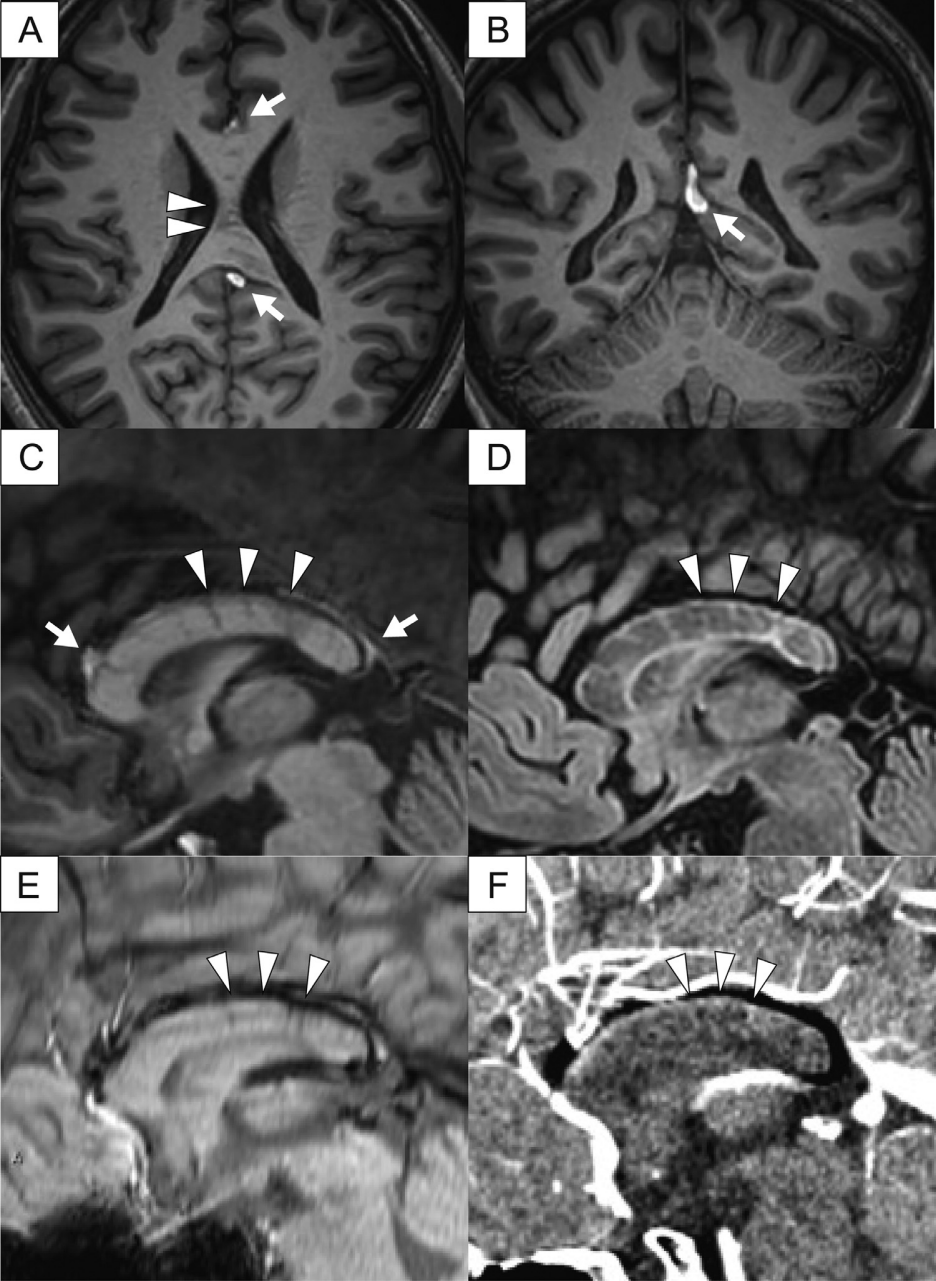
Imaging studies. Axial, coronal and sagittal magnetic resonance imaging of the brain shows a curvilinear hyperintense lesion along the somewhat hypoplastic corpus callosum on magnetization-prepared rapid acquisition with gradient echo (MPRAGE) (A, B, C), indicating a pericallosal lipoma of curvilinear type (arrow). Within the corpus callosum, “caterpillar sign” (arrowheads) are seen with low signal on MPRAGE (A, C), high signal on fluid-attenuated inversion recovery (D), low signal on susceptibility-weighted imaging (SWI) (E), and slightly contrast-enhanced on postcontrast computed tomography (F), respectively.
